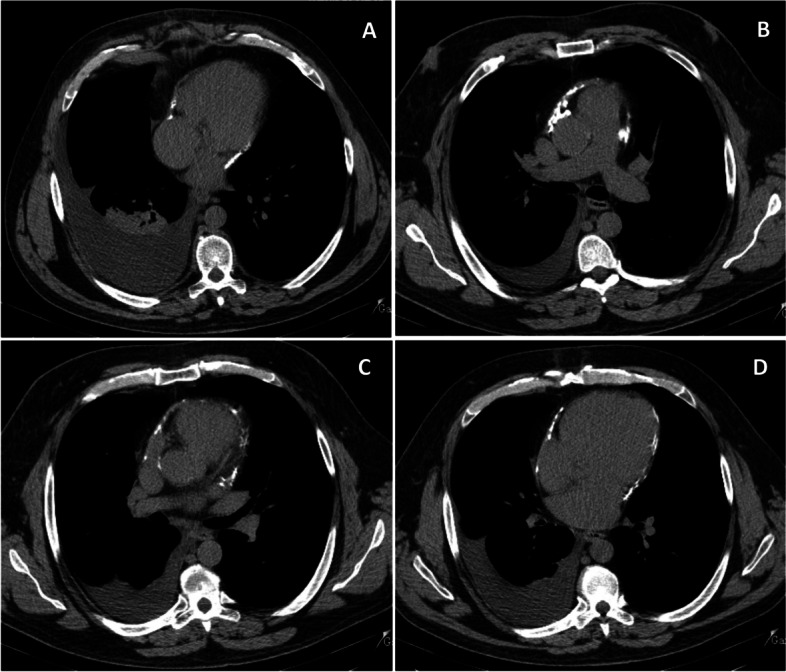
Chest computed tomography performed during hospital stay. A Right pleural effusion (6 cm) causing right lower lobe collapse. B, C, D Pericardial thickening with extensive calcified pericardial plaques
